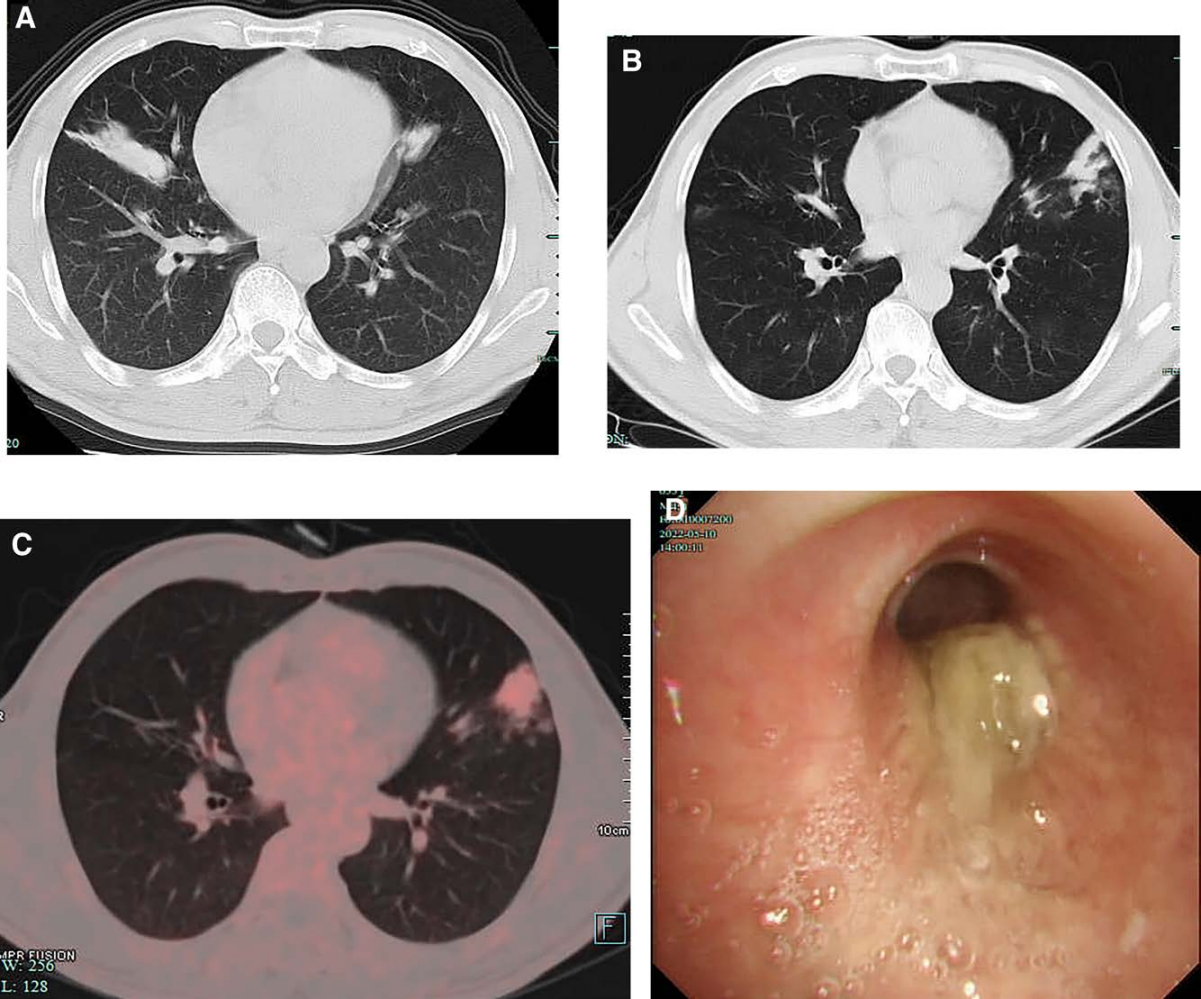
(A) Chest computed tomography (CT) showed gloved finger sign, consolidation in the middle lobe of the right lung and upper lobe of the left lung 6 years ago. (B) A patchy of consolidation, infiltrates and gloved finger sign were seen in the left lingular lobe on Chest CT. (C) PET/CT showed mild metabolic activity with standard uptake value (SUV max 3.5) in the left lingular lobe which consistent with inflammation. (D) Bronchoscopy showed mucosal swelling and thick white mucous plugs in the left lingular bronchus which could be removed partially by suctioning.
